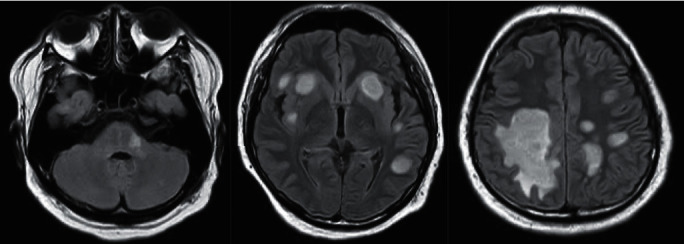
Axial FLAIR brain MRI of an 18-year-old male with ADEM, who presented with tetraparesis, predominantly on the left side. There were multiple hyperintense lesions on the left cerebellar peduncle, left cerebellum, and bilateral cerebral white matter.
